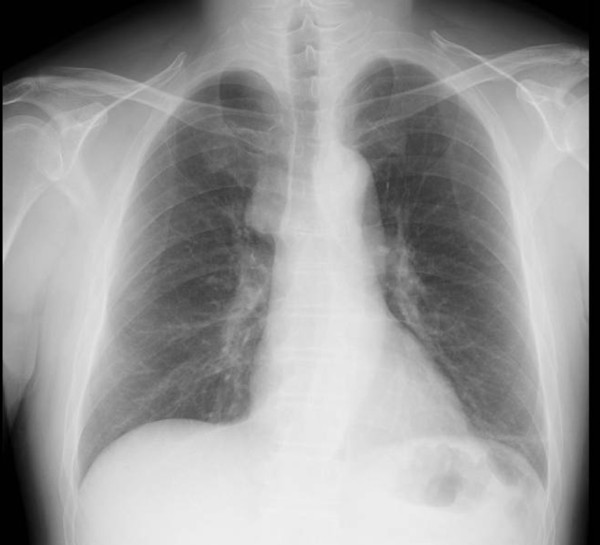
Chest x-ray showing a solid mass with a clear border at the right hilum and a negative silhouette sign for the right first arch.
